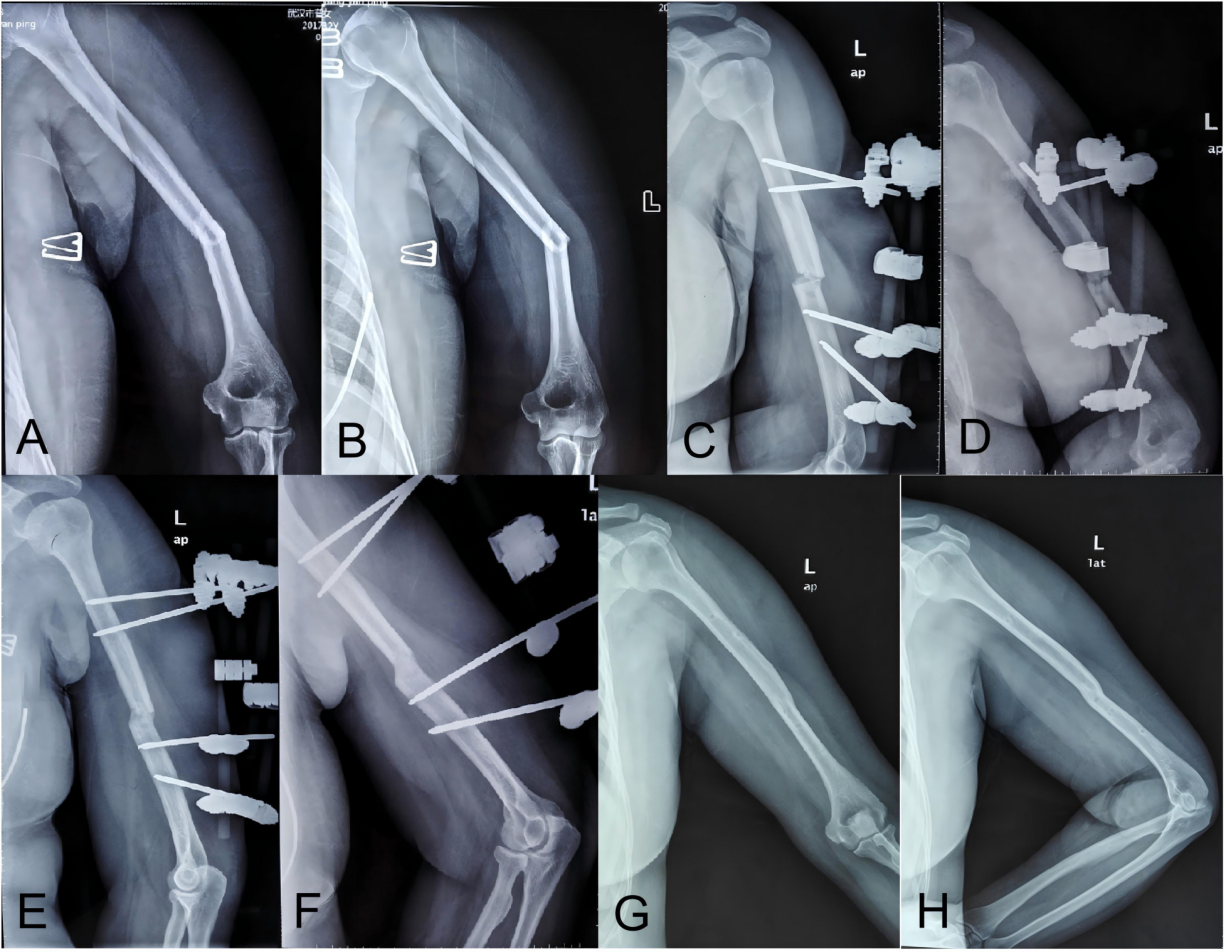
32-year-old female with closed left humeral shaft fracture (AO/OTA A3). (A,B) Initial anteroposterior and lateral radiographs. (C,D) Post-closed reduction EF. (E,F) 11-week follow-up showing union; fixator removed. (G,H) Final follow-up radiographs showing cortical continuity and medullary canal patency.
